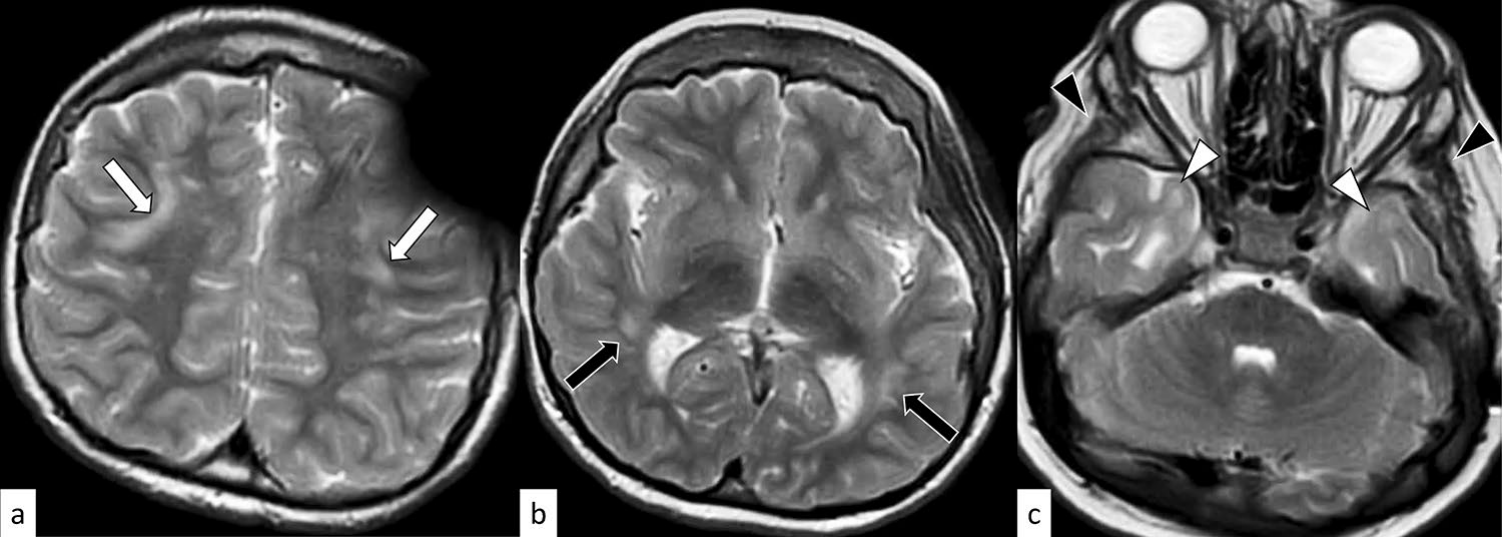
An 11-year-old boy with congenital DM1. T2-weighted axial images (a–c) show hyperintensity in the white matter of the bilateral frontal lobes (a, white arrows), trigone of the bilateral temporal lobes (b, black arrows), and the bilateral temporal poles (c, white arrowheads). Atrophy of the bilateral temporalis muscles is observed (c, black arrowheads)
